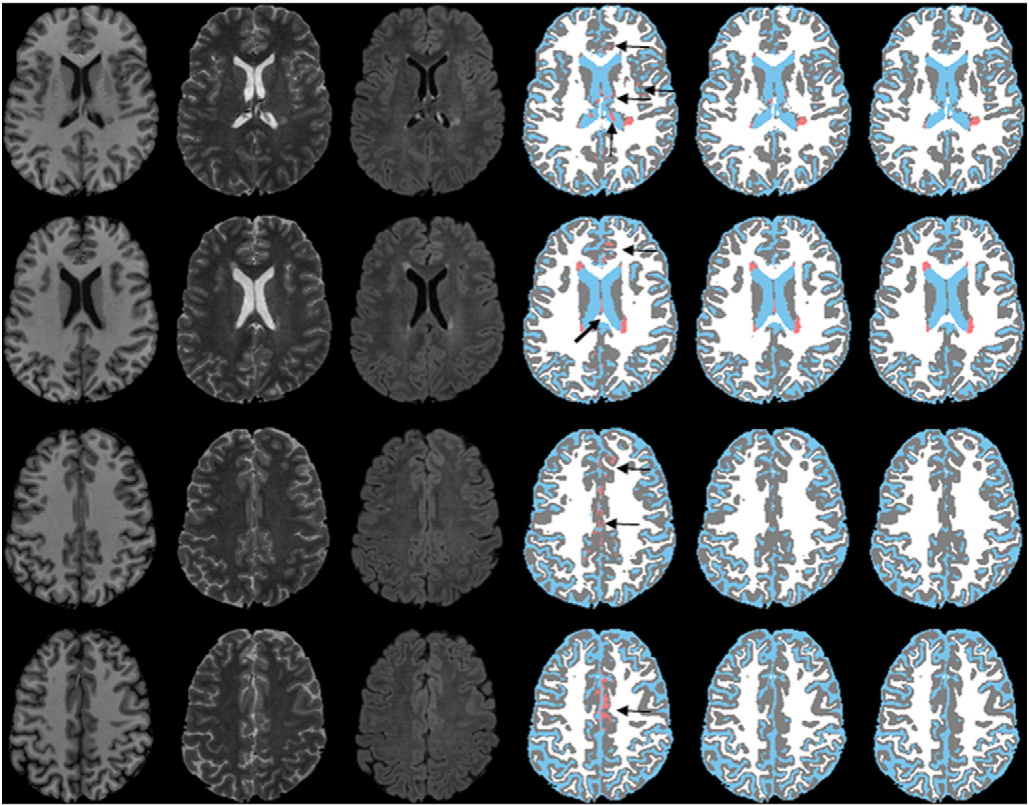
Results of various steps for minimizing the false classifications at different cross-sections from one subject. First column: T1; second column: T2; third column: FLAIR; fourth column: images obtained with the Parzen classification on which false classifications are pointed by arrows; fifth column: images following the minimization of false classifications; and sixth column: images with corrected lesions. Color scheme is same as Fig. 2.
